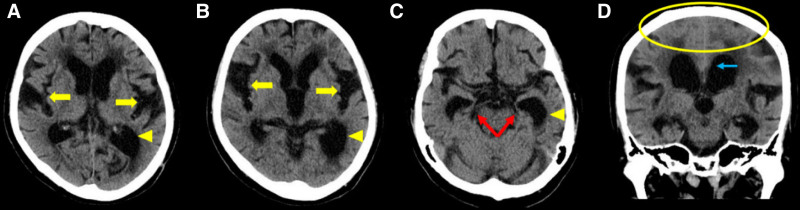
Preoperative computed tomography demonstrating ventricular enlargement with an Evans index of 0.33. (A, B) Horizontal sections showing widening of the Sylvian fissure (yellow arrow) and posterior-dominant enlargement of the left lateral ventricle (yellow arrowhead). (C) A different horizontal section showing the posterior-dominant enlargement of the left lateral ventricle (yellow arrowhead), with marked bilateral hippocampal atrophy (red arrow). (D) Coronal section demonstrating a positive “disproportionately enlarged subarachnoid space hydrocephalus” (DESH) sign (within the yellow box) and a callosal angle of <90° (blue arrow). DESH = disproportionately enlarged subarachnoid space hydrocephalus.
